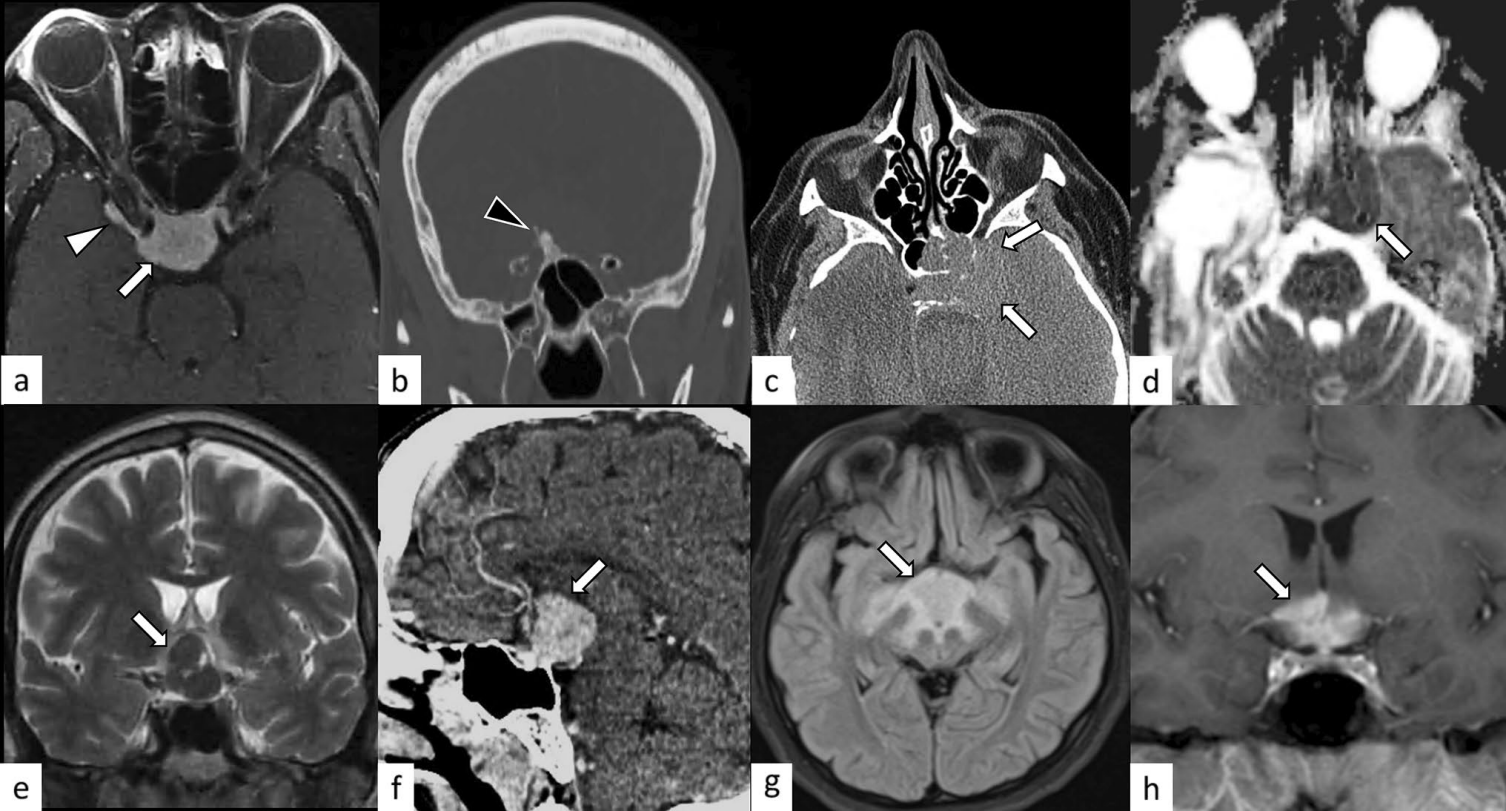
a, b A 49-year-old man with meningioma. Post-contrast fat-suppressed T1-weighted image shows homogeneously enhanced suprasellar mass (a, arrow). Dural-tail sign (a, white arrowhead) and hyperostosis (b, black arrowhead) are observed. c, d A 60-year-old woman with lymphoma. Non-enhanced CT shows an infiltrative hyperdense suprasellar mass (c, arrowheads), which shows restricted diffusion with low apparent diffusion coefficient (d, arrow). e, f A 51-year-old man with pituicytoma. T2-weighted coronal image shows a suprasellar well-defined mass with homogeneous hypointensity and small cystic areas (e, arrow). Contrast-enhanced CT shows homogeneous enhancement (f, arrow). g, h A 14-year-old girl with Rosai–Dorfman disease. FLAIR image shows an infiltrative suprasellar mass with an ill-defined margin, involving the midbrain and bilateral thalami (g, arrow). Post-contrast T1-weighted image shows heterogeneous and strong enhancement (h, arrow)
